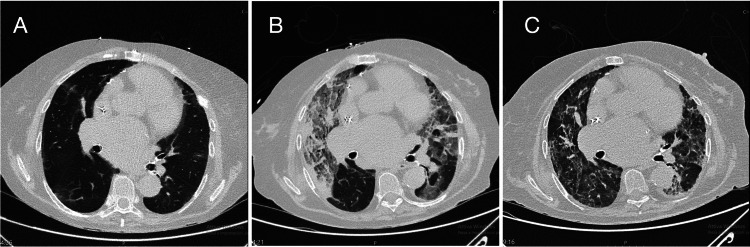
Evolution of non-enhanced axial CT scans at the lung bases. (A) Baseline scan (20 months prior) showing chronic interstitial changes. (B) Admission scan confirming diffuse ground-glass opacities and consolidation. (C) Day 10 scan showing significant radiological clearance.
